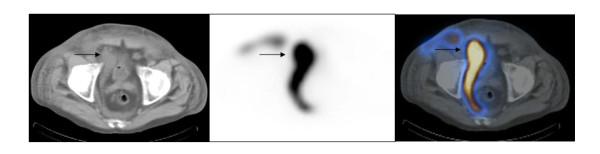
Axial images of SPECT/CT of pelvis. The images show a urine leak (arrows) in the right pelvic cavity next to the urinary bladder extending to the right inguinal canal and abdominal wall anteriorly and the presacral region posteriorly.
